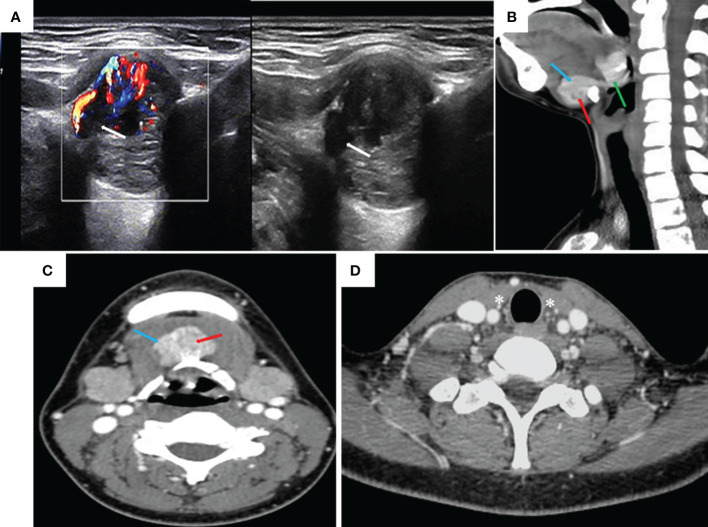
Color Doppler ultrasound and contrast-enhanced CT. (A) Ectopic thyroid and carcinoma nodule at the floor of the mouth (very hypoechoic area shown by the white arrow). (B, C) Ectopic thyroid gland at the base of the tongue, ectopic thyroid gland at the base of the mouth, and a single cancer nodule (the green arrow is the thyroid gland at the base of the tongue, the blue arrow is the thyroid gland at the base of the mouth, and the red arrow is a cancer nodule). (D) No thyroid gland in the normal position of the neck (white * area).
